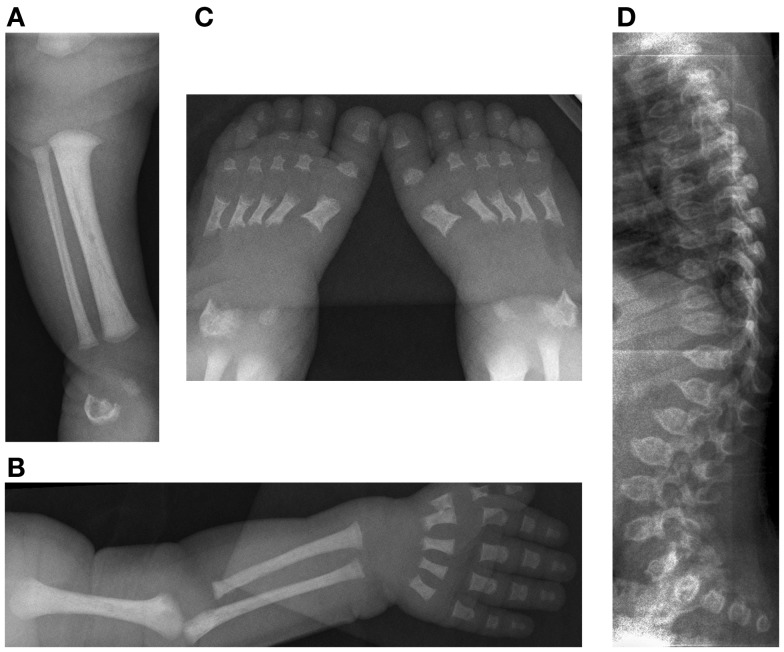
Case 2, Initial skeletal survey at 9 days of life (A) [10/09/08] demonstrates lack of visualization of the distal femoral and proximal tibial ossification centers, (B) relatively short long bones of the left upper extremity and (C) feet with metaphyseal cupping and irregular ossification of the talus and calcaneus. The degree of platyspondyly (D) is less than that of her sibling on lateral spine radiographs.
